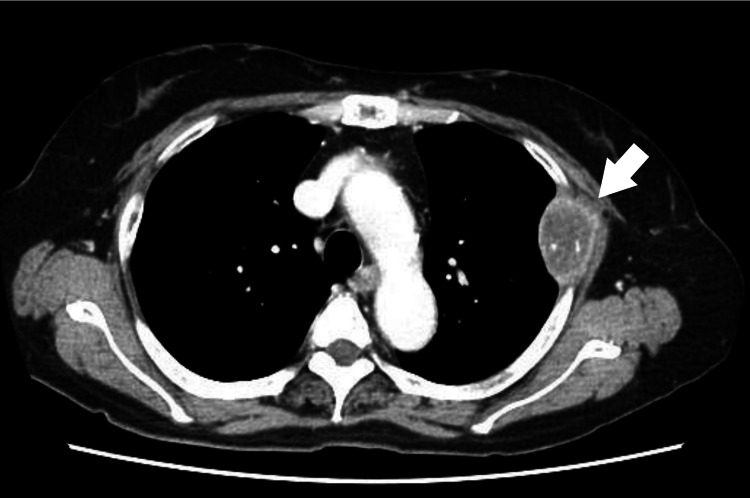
CT showing a destructive mass in the left fourth rib with ring-like enhancement (arrow).
